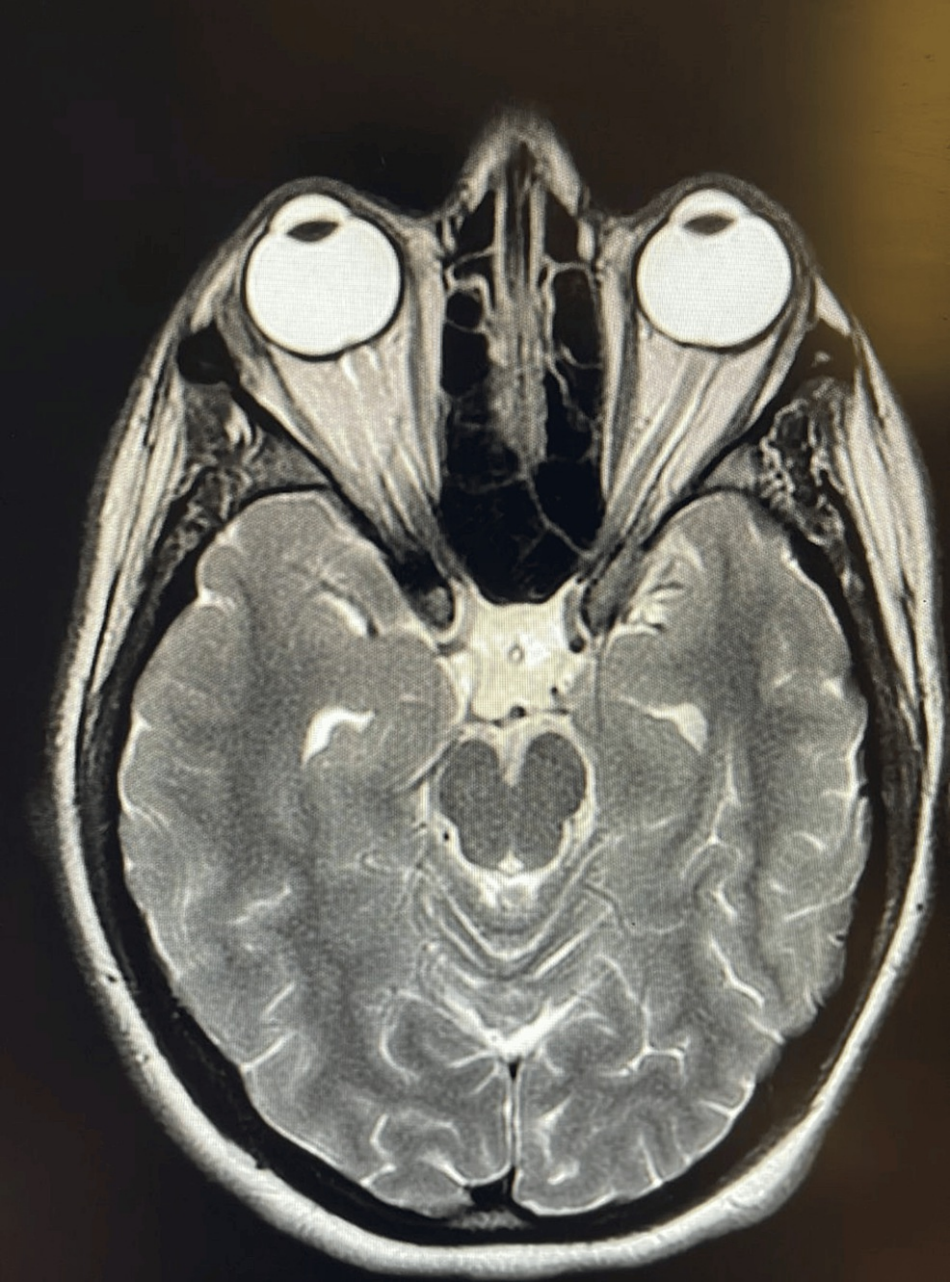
Axial T2 weighted MRI showing a distended optic nerve sheath and flattening of the globe at the disc
